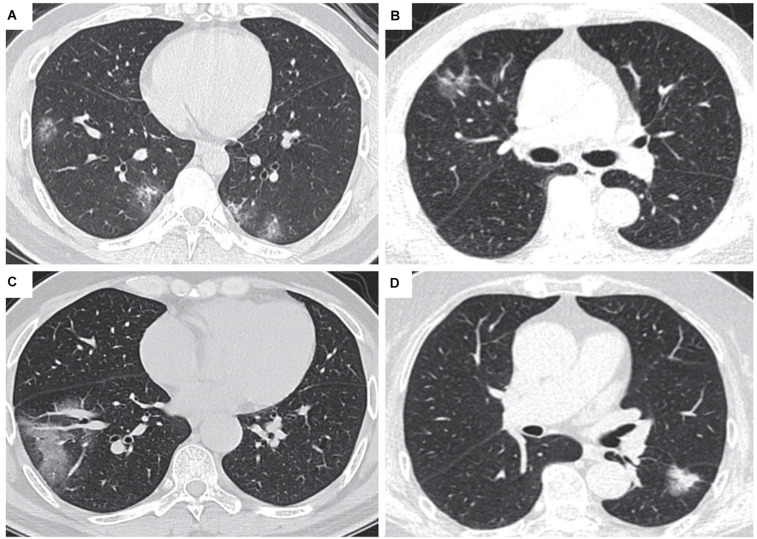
CT images of lung cancer patients and COVID-19 (Transl Lung Cancer Res.2020 Aug;9(4):1516-1527. doi: 10.21037/tlcr-20-892). (A) CT scan of a patient diagnosed with COVID-19: The lesion is GGO, and there is no sign of pleural retraction or vascular convergence. (B) CT scan of a patient diagnosed with lung cancer, with pleural retraction and cystic change. (C) CT scan of a patient diagnosed with COVID-19, the lesion showed patchy turbidity, irregular shape, and no pleural retraction. (D) CT scan of a patient diagnosed with lung cancer, with pleural retraction, lobulated sign, and spiculate protuberance.
